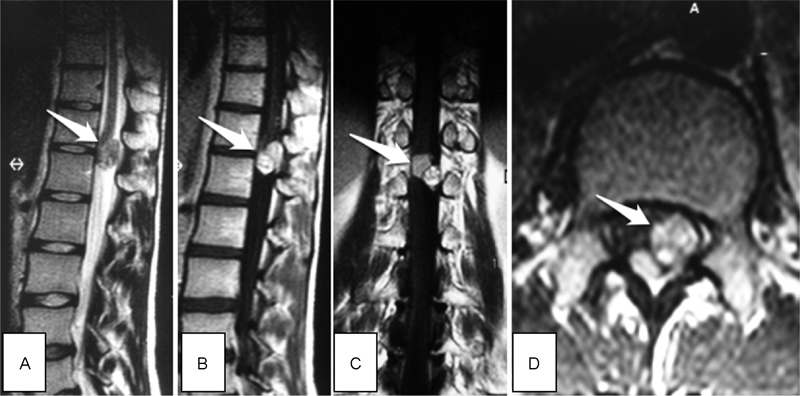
First manifestations of the tumor in 2002 in MR imaging (the arrow points to the tumor). (
